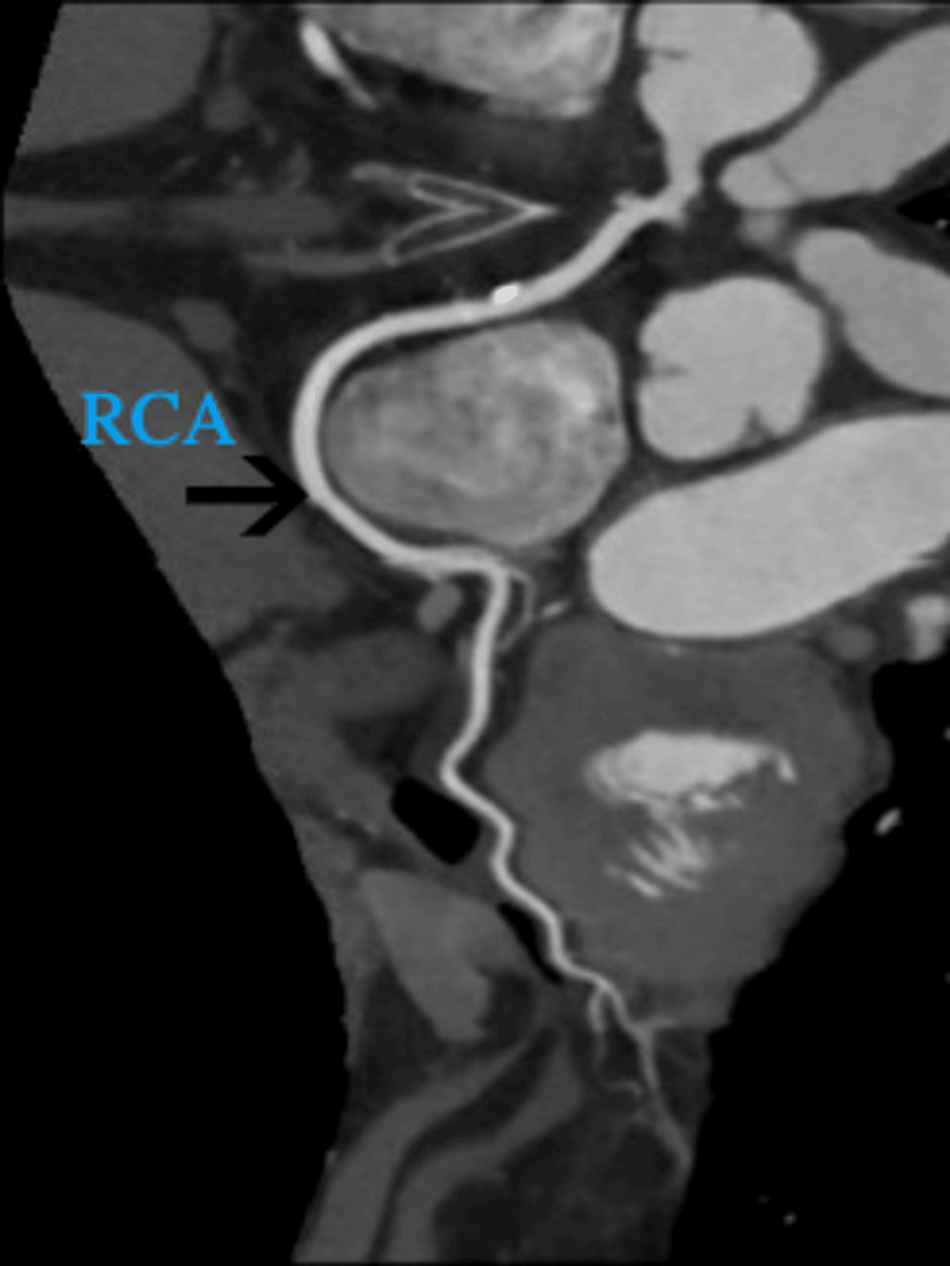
Coronary computed tomography angiography (CCTA) image showing dominant and large right coronary artery (RCA)
